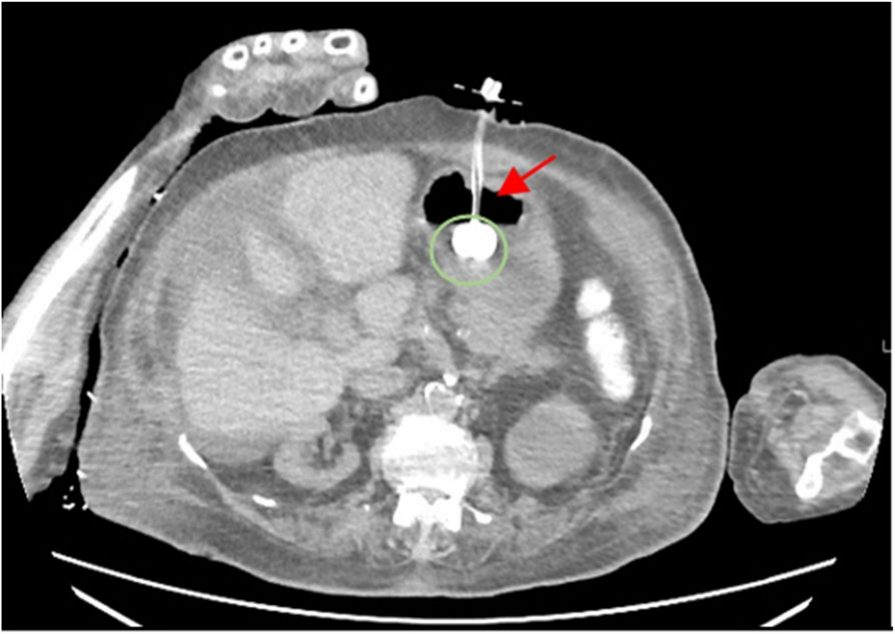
A non-enhanced CT scan of the PEG tube three days post-enjection, showcasing a patent ballon (green circle), a clear stomach wall devoid of necrosis or hematoma, and an absence of any bleeding (red arrow)
